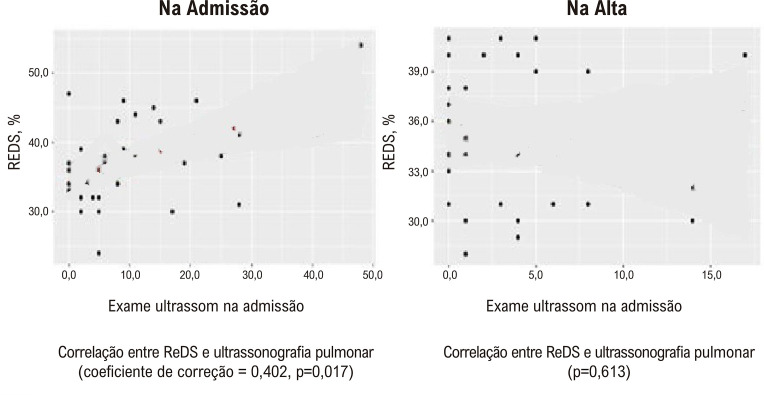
Relação de correlação entre ReDS (%) e ultrassonografia pulmonar (soma das linhas B).
